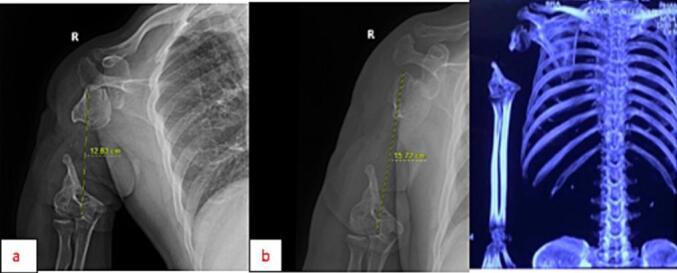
X-ray of the patient's right shoulder not bearing weights (figure a), bearing weights (figure b) and CT-scan of his right arm.
